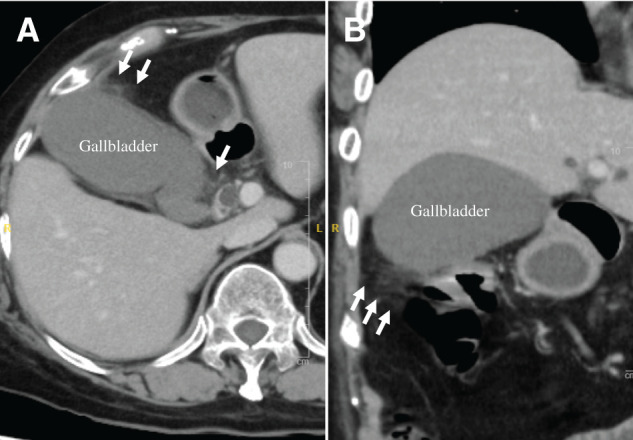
Abdominal contrast-enhanced CT findings. CECT shows gallbladder distension and pericholecystic inflammatory changes (arrows). The gallbladder wall shows no contrast enhancement, indicating gangrenous cholecystitis. (A) Axial image. (B) Coronal image. CECT, contrast-enhanced CT
